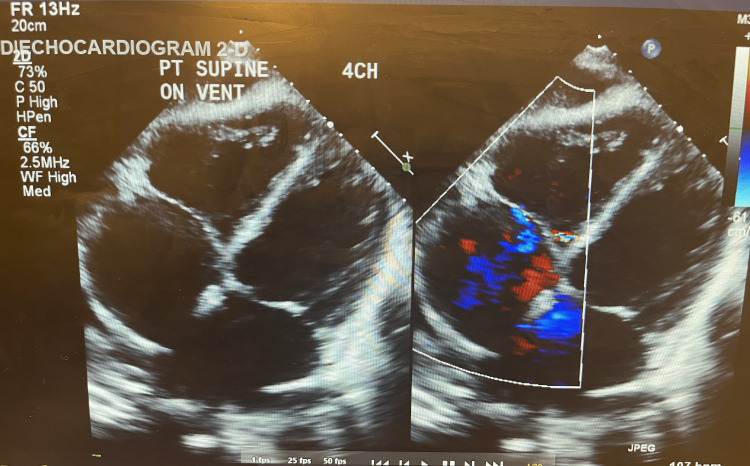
Two-dimensional echo significant for four-chamber dilation with mild global hypokinesis and a reduced LVEF of 40%-45% echo, echocardiography; LVEF, left ventricular ejection fraction
